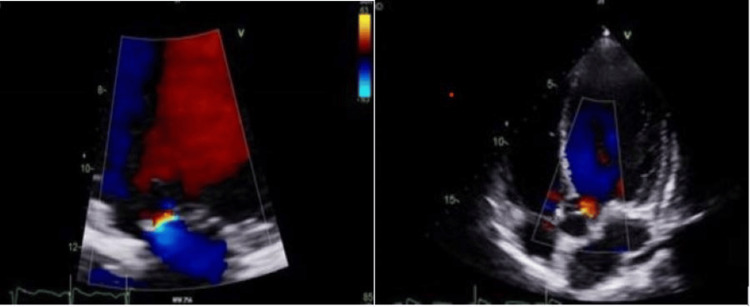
Transthoracic echocardiogram a: trace mitral regurgitation; b: trace aortic regurgitation
